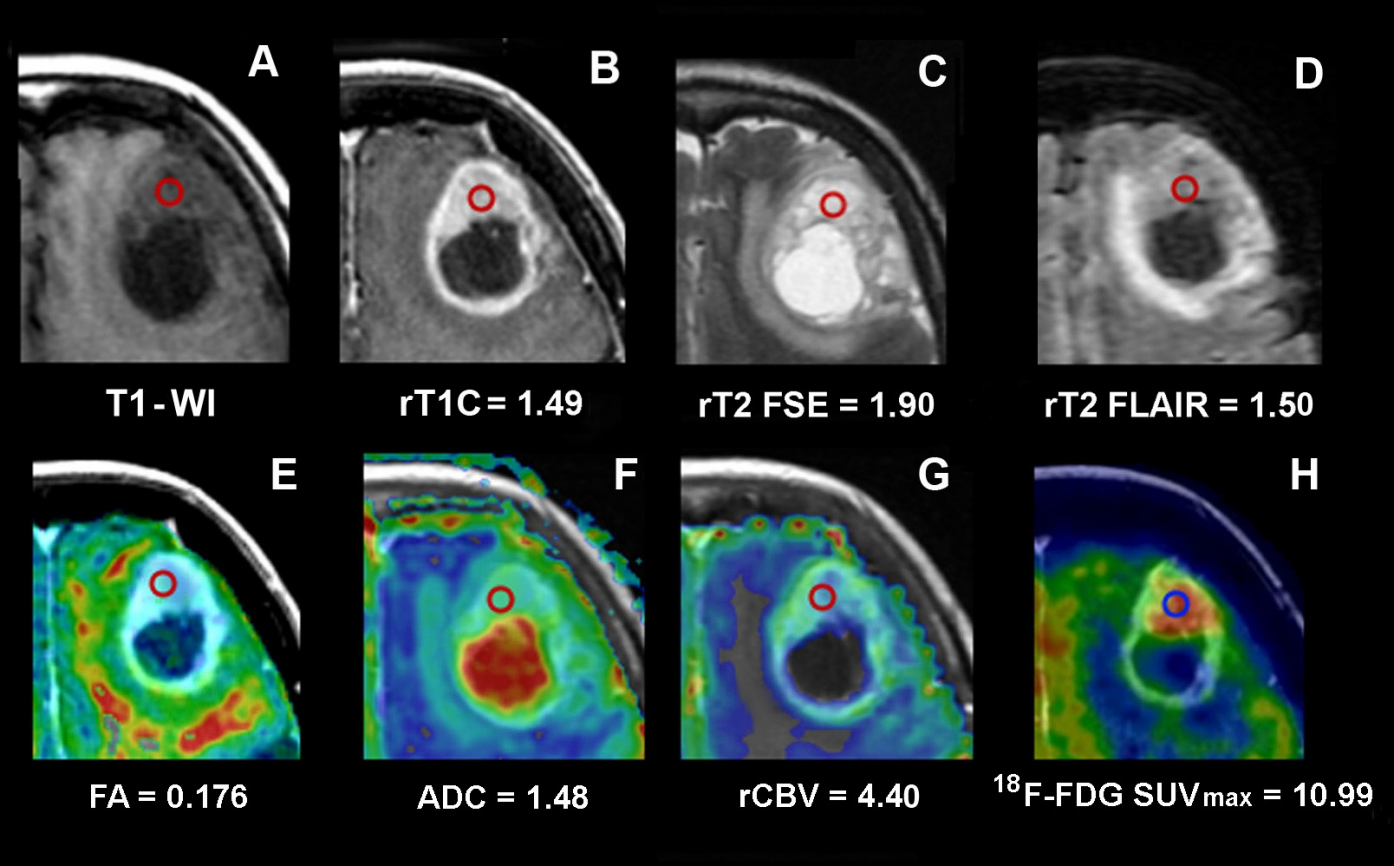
Conventional, advanced MRI and ^18^F-FDG PET/CT/MR fusion image of a frontal GB Images of a frontal GB in a 77-year-old woman (Case CTO3) and values from the ROI in the CE. The ROI is indicated by the red circle. (A) T1 weighted MRI. (B) T1C MRI and rT1C value. (C) T2 FSE MRI and rT2 FSE value. (D) T2 FLAIR MRI and rT2 FLAIR value. (E) FA color map fused with axial T1C MRI and FA value. (F) ADC color map fused with axial T1C MRI and ADC value. (G) rCBV color map fused with axial T1C MRI and rCBV value. (H)
